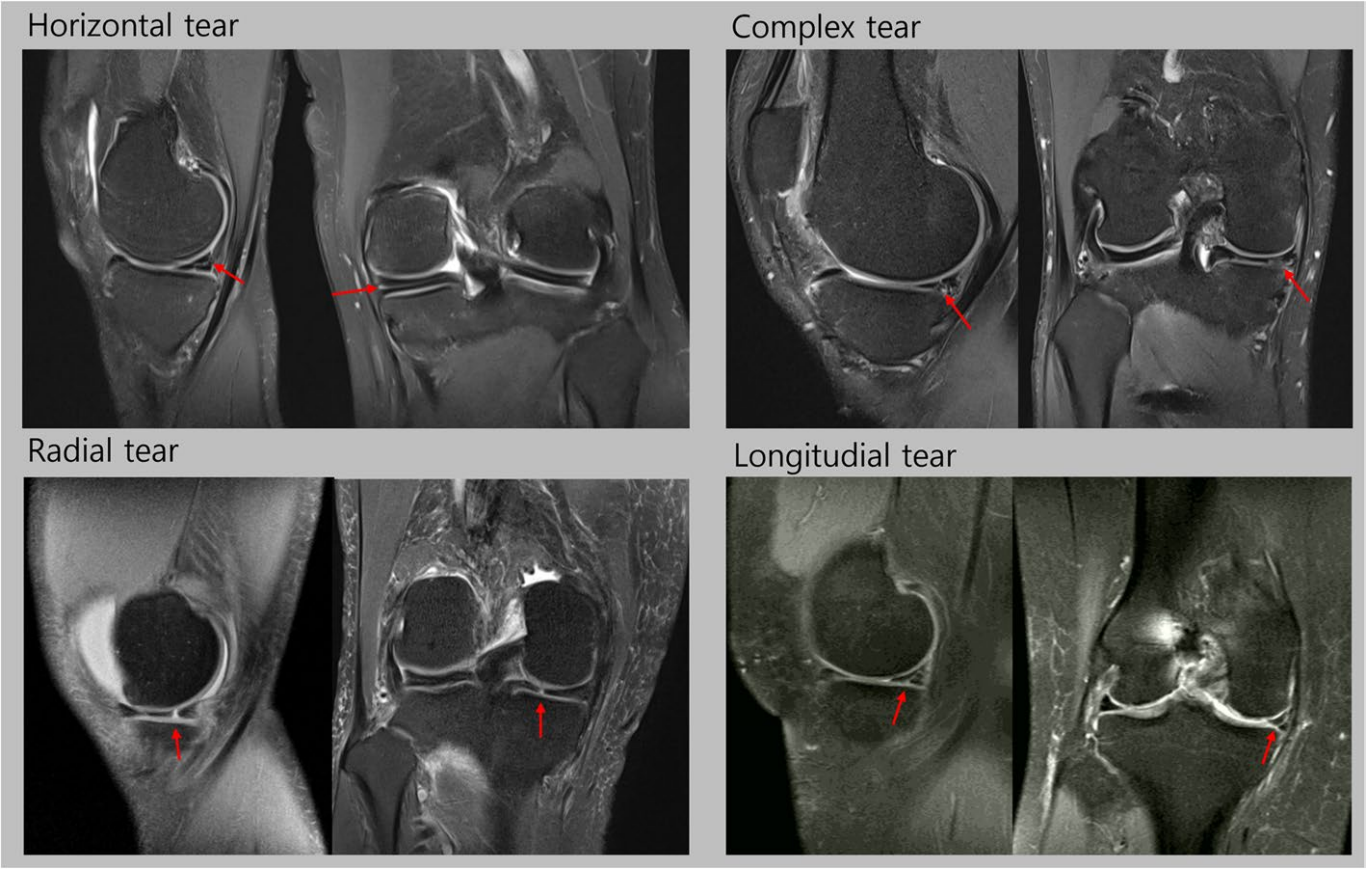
Representative magnetic resonance images of each type of meniscus tear
